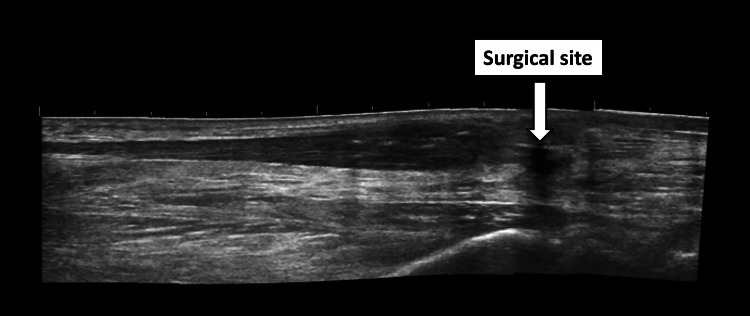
Ultrasound examination Ultrasound examination at five months postoperatively, displaying satisfactory healing of the surgical site.
